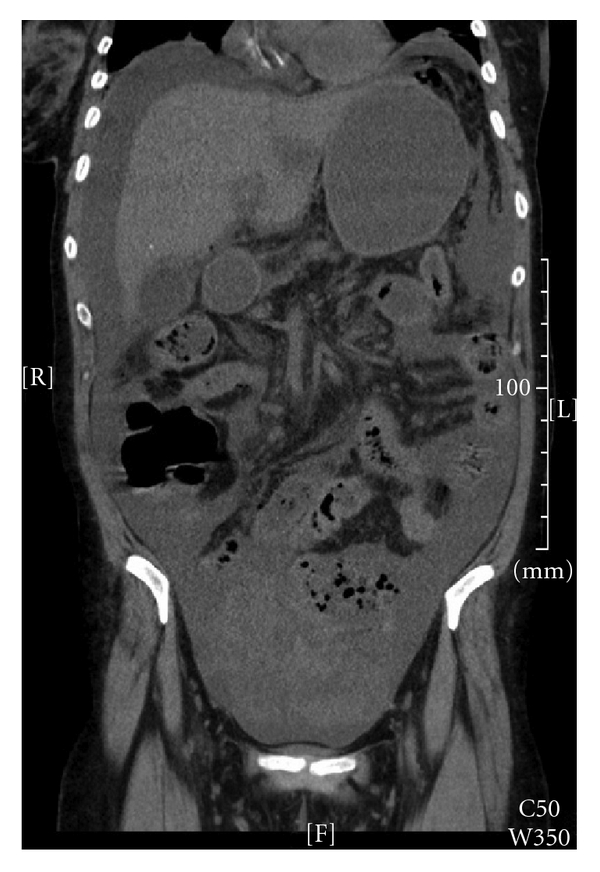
CT showing mass-like cystic structure in pelvis with air bubbles.
